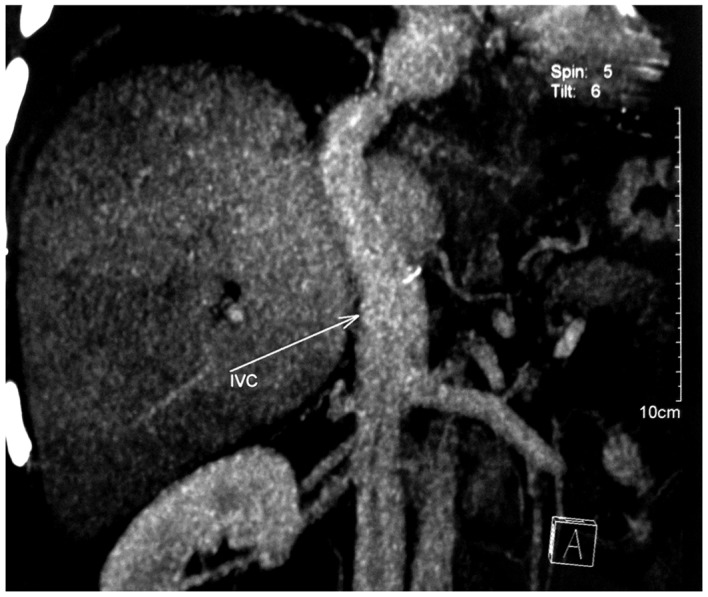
Abdominal computed tomography (CT) and CT angiography (CTA) results showed that no significant stenosis or occlusion existed in the hepatic vein, inferior vena cava (IVC) or portal vein of the patient.
